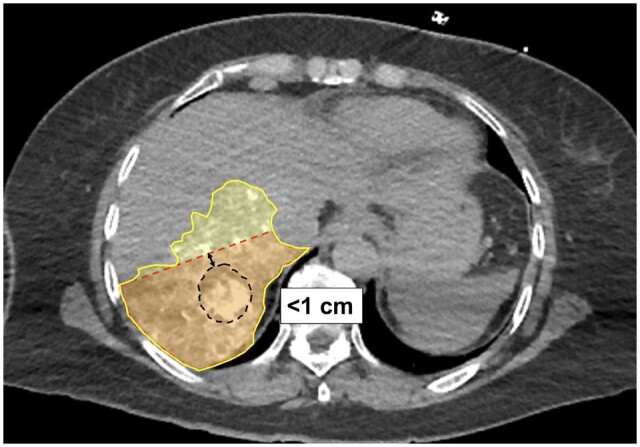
Tumours located within 1 cm of a watershed junction were significantly associated with underestimated volumetric measurements based on Couinaud anatomic method. In this case, a 3.9-cm HCC tumour (black dashed line) was seen centrally within segment 7, although it is within 1 cm of the watershed regions of segments 6/7 and 7/8 (Patient 1 from Supplementary Table S1). The tumoural angiosome acquired by pre-TARE treatment Angio-CT (yellow area) extended from segment 7 (as defined by conventional Couinaud landmarks, orange-red area) into segment 8.
